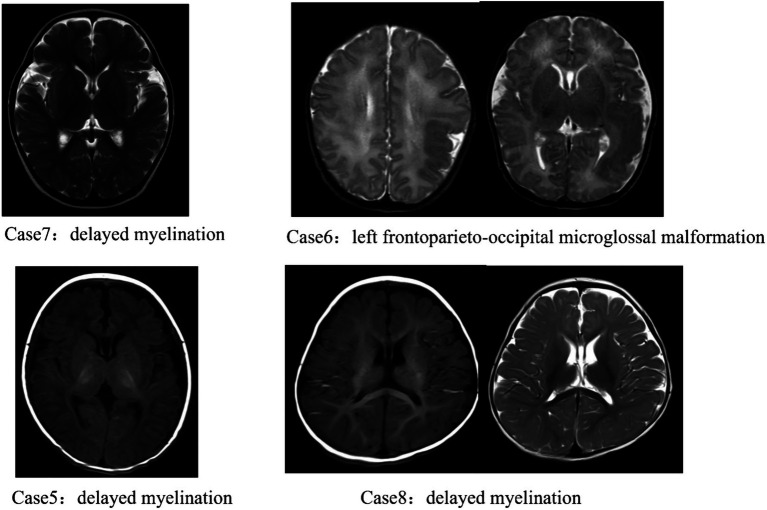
Abnormal cranial magnetic resonance imaging results of 4 pediatric patients.
